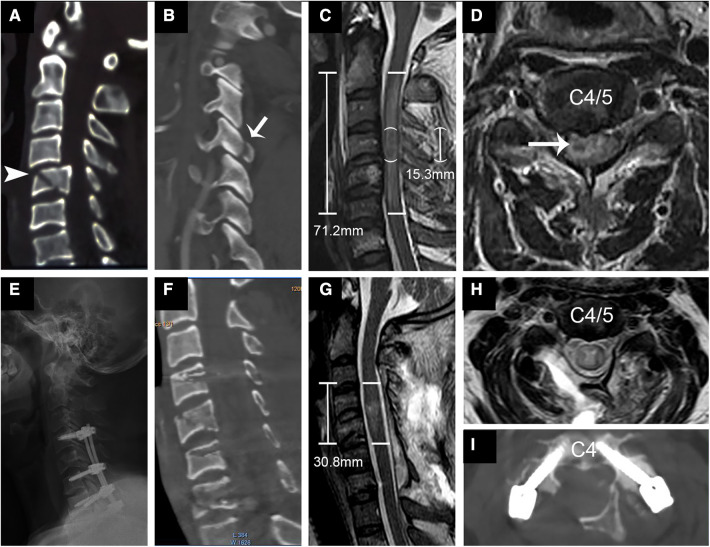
Representational preoperative (A–D) and postoperative (E–I) images of a 44-year-old male patient who sustained a C type fracture and spinal cord injury. His AMS was 3 and AIS grade A. Sagittal subaxial CT indicated C4 translation rotation injury (A) accompanied by fracture of the right inferior articular process (B, short arrow) and C5 teardrop fracture (arrowhead). MRI (C,D) showed SCI with an IMLL of 71.2 mm and intramedullary hemorrhage (long arrow) 15.3 mm in length. The subarachnoid space (SAS) was absent from C3 to C5 (C). Laminoplasty of C3–7 and pedicle screw fixation from C4–C7 were performed in this patient 14 h after trauma. Postoperative x-rays (E) and CT (F,I) showed good screw positioning and significant enlargement of the osseous spinal canal. Postoperative MRI indicated an IMLL of 30.8 mm (G) and successful decompression (G,H) 12 days after trauma. One year after injury, the patient paralysis recovery with an AMS of 58 and AIS grade C.
